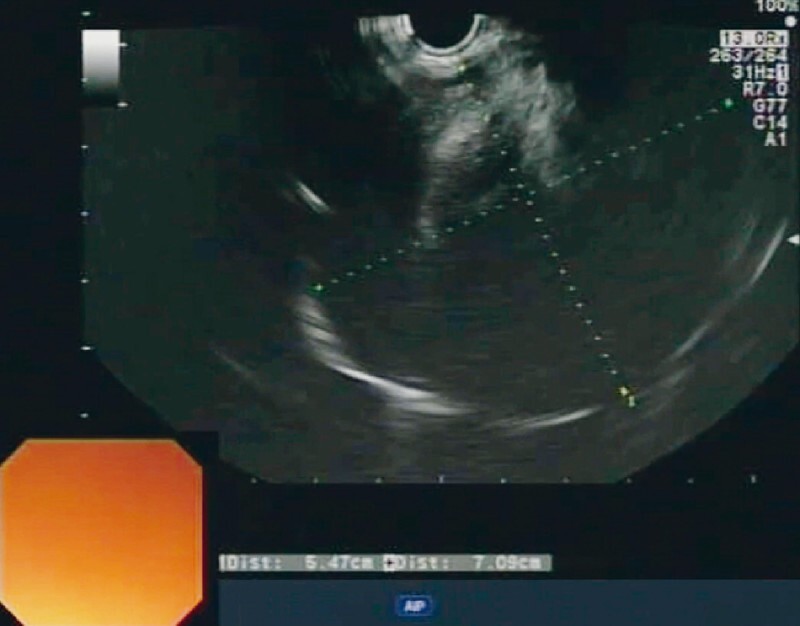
Endoscopic ultrasound view of the lesion showing a 7-cm hypoechoic mass.
